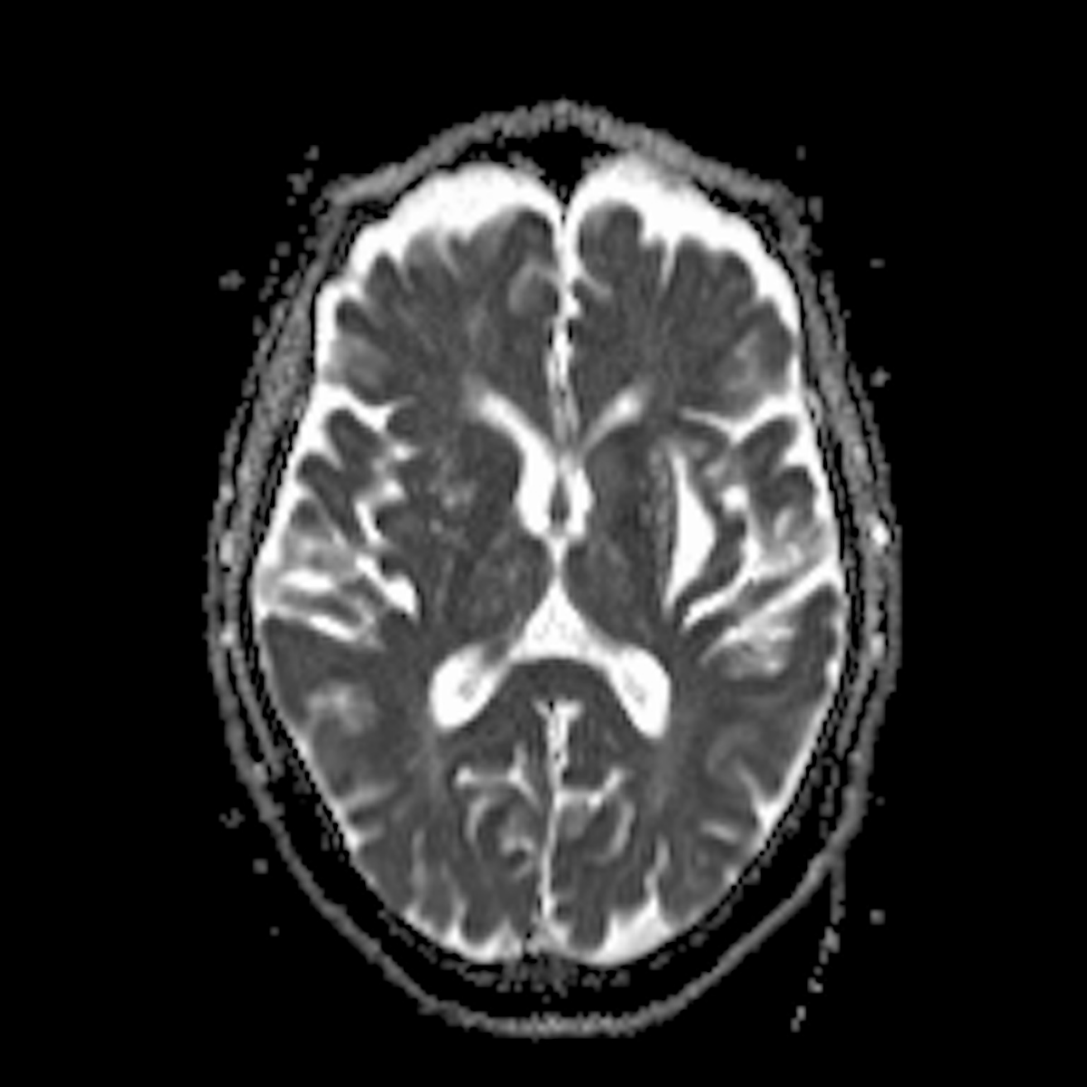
Magnetic resonance imaging (MRI) MRI without apparent diffusion coefficient (ADC) correlate.
